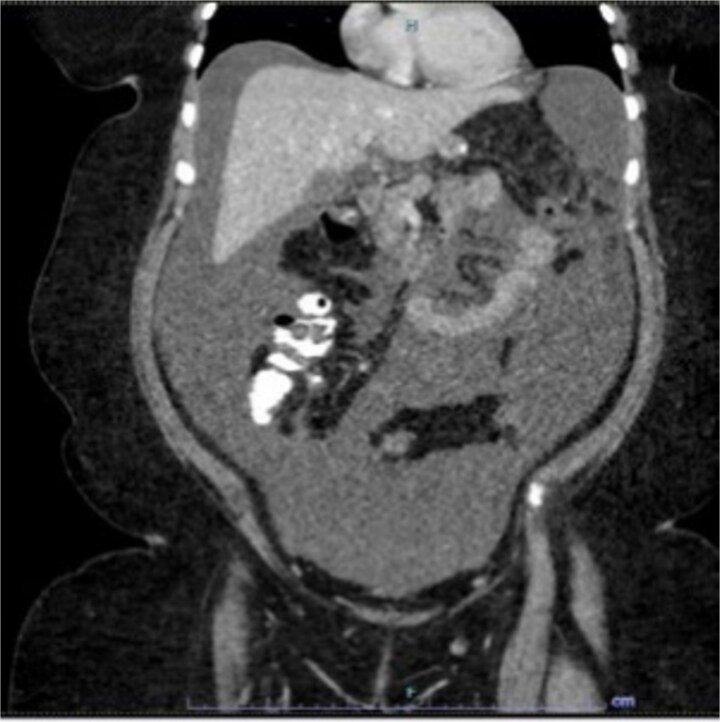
CT scan showing large volume abdominal ascites at post operative day 51.
